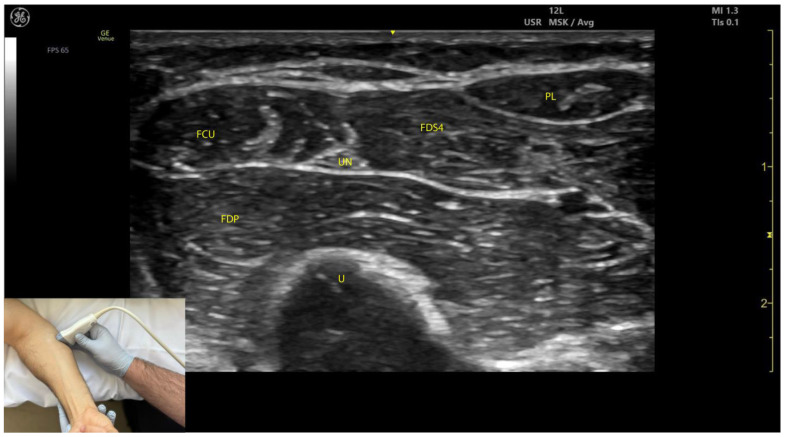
Ultrasound anatomy of the flexor digitorum superficialis (FDS4) with key landmarks: FDS4—flexor digitorum superficialis digit 4; PL—palmaris longus; FCU—flexor carpi ulnaris; U—ulna; UN—ulnar nerve; FDP—flexor digitorum profundus.
